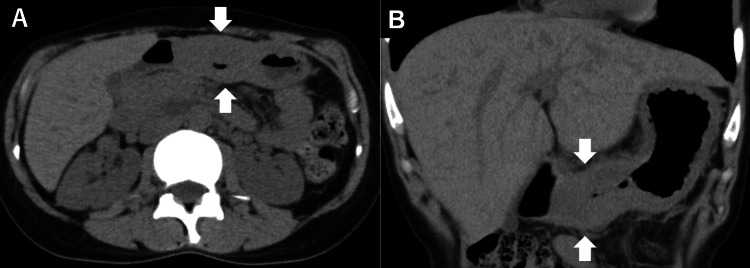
Computed tomography (CT) finding. CT showing marked thickening of the gastric wall, predominantly in the antrum (arrows), suggestive of infiltrative gastric malignancy. Axial (A) and coronal (B) views of the CT scan.
